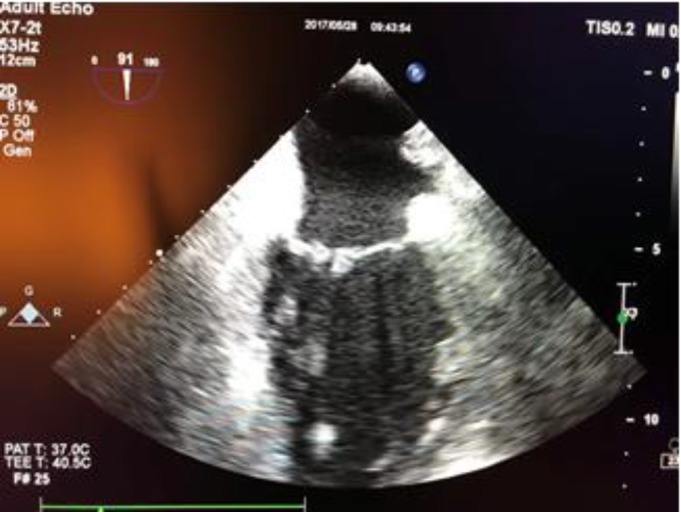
Transesophageal echocardiography (TEE) showed two small vegetations on the tip of right cusp and non-coronary cusp of bicuspid aortic valve
